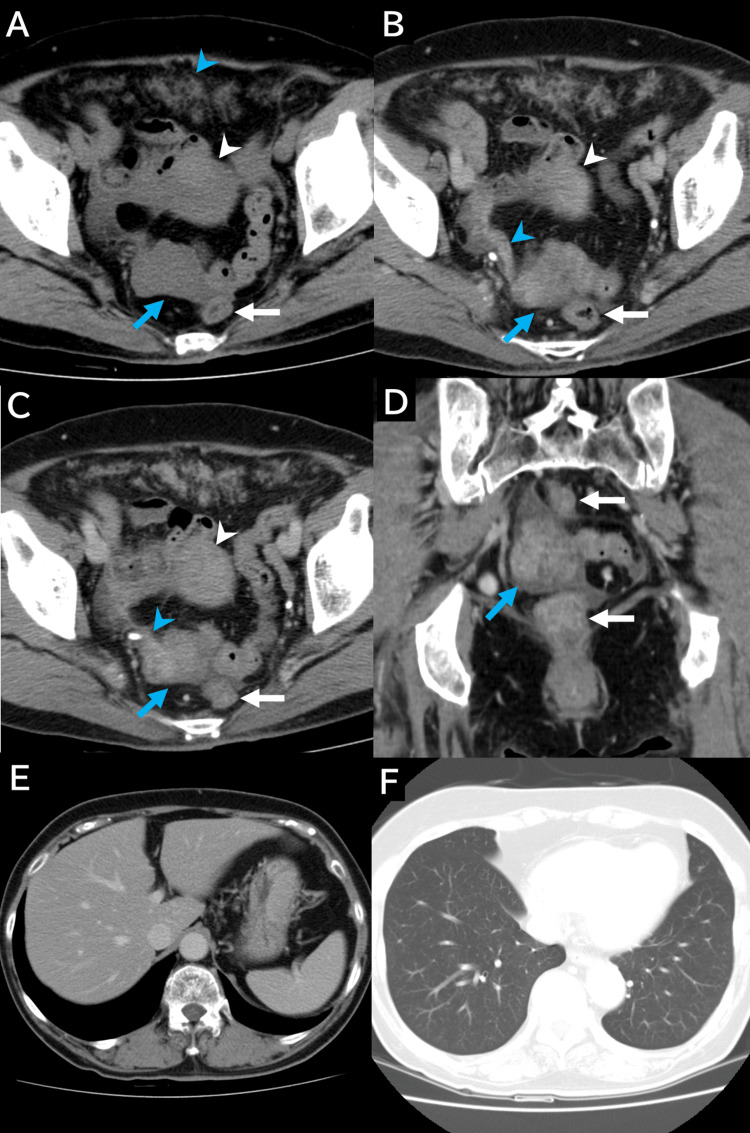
CT findings (A) On unenhanced axial CT, a 40 × 35 mm round mass (blue arrow) with homogeneous internal density equivalent to the uterus (white arrowhead) is observed on the right side of the rectum (white arrow). The mass is separated from the uterus (white arrowhead). Numerous ill-defined peritoneal nodules are present in the omentum (blue arrowhead). (B and C) On contrast-enhanced axial CT, the mass (blue arrow) located on the right side of the rectum (white arrow) shows mildly heterogeneous enhancement similar to that of the uterus (white arrowhead). The right ovarian vein (blue arrowhead) is continuous with the mass. (D) On contrast-enhanced coronal CT, the mass demonstrates a heterogeneous internal structure with patchy enhancement (blue arrow). The rectum is adjacent to the mass (white arrow). (E) On contrast-enhanced axial CT, no liver metastases or peritoneal nodules around the liver are observed. (F) No metastatic tumors are present in the lungs. CT: computed tomography
